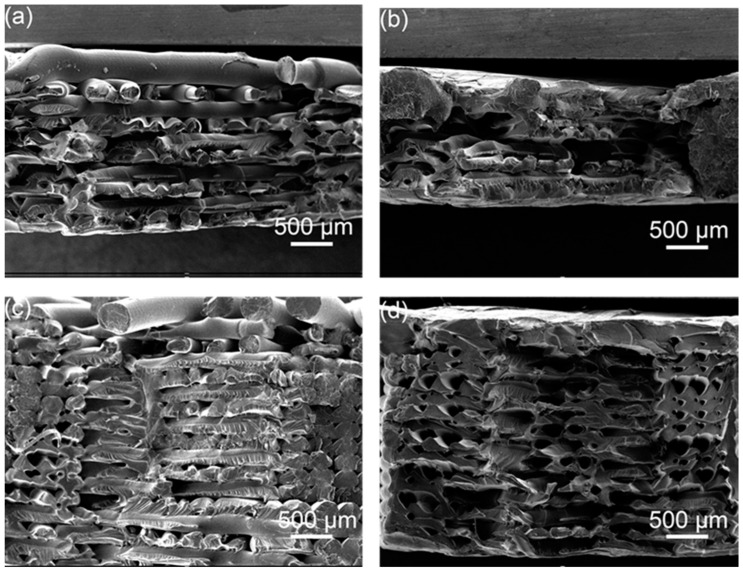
Cross-sectional view of the tensile sample, (a) 2 mm original, (b) 2 mm ultrasonically strengthened sample, (c) 3.5 mm original, (d) 3.5 mm ultrasonically strengthened sample.
